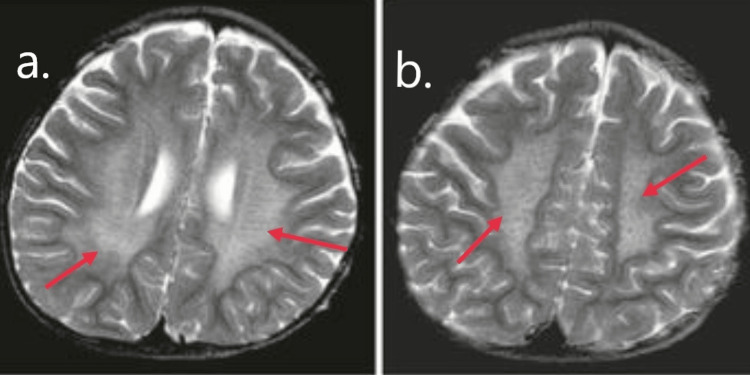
Red arrows in both images a. and b. are of MLD of periventricular white matter These T2-weighted MRI images show symmetrical hyperintensities in bilateral periventricular and cortical white matter is suggestive of Metachromatic leukodystrophy (MLD).
